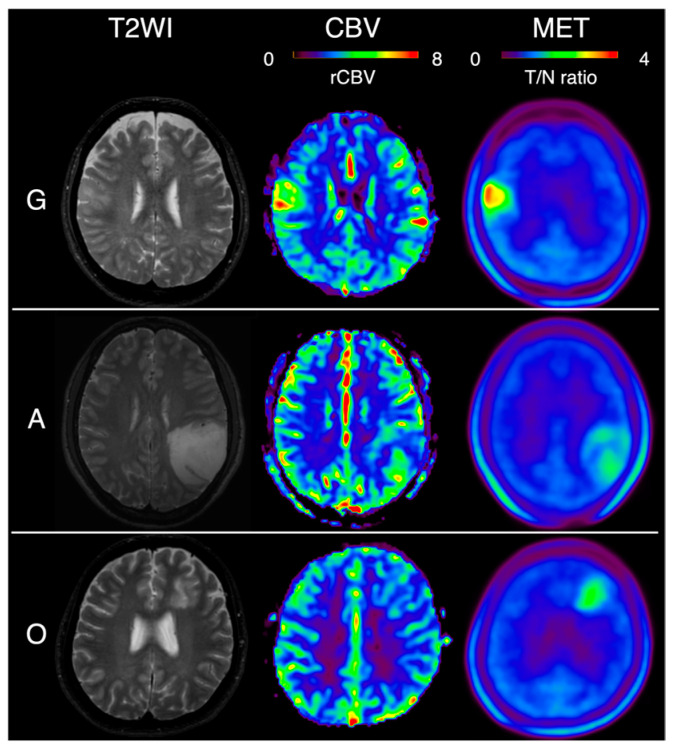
T2-weighted image (T2WI), relative cerebral blood volume (rCBV), and methionine (MET) PET imaging in typical cases of each glioma subtype. G: glioblastoma isocitrate dehydrogenase (IDH)-wildtype, A: astrocytoma, IDH-mutant, O: oligodendrogliomas, IDH-mutant, and 1p/19q-codeleted. Color map scales: CBV, rCBV-to-white matter ratio up to 8; MET, tumor-to-normal (T/N) ratio up to 4.
